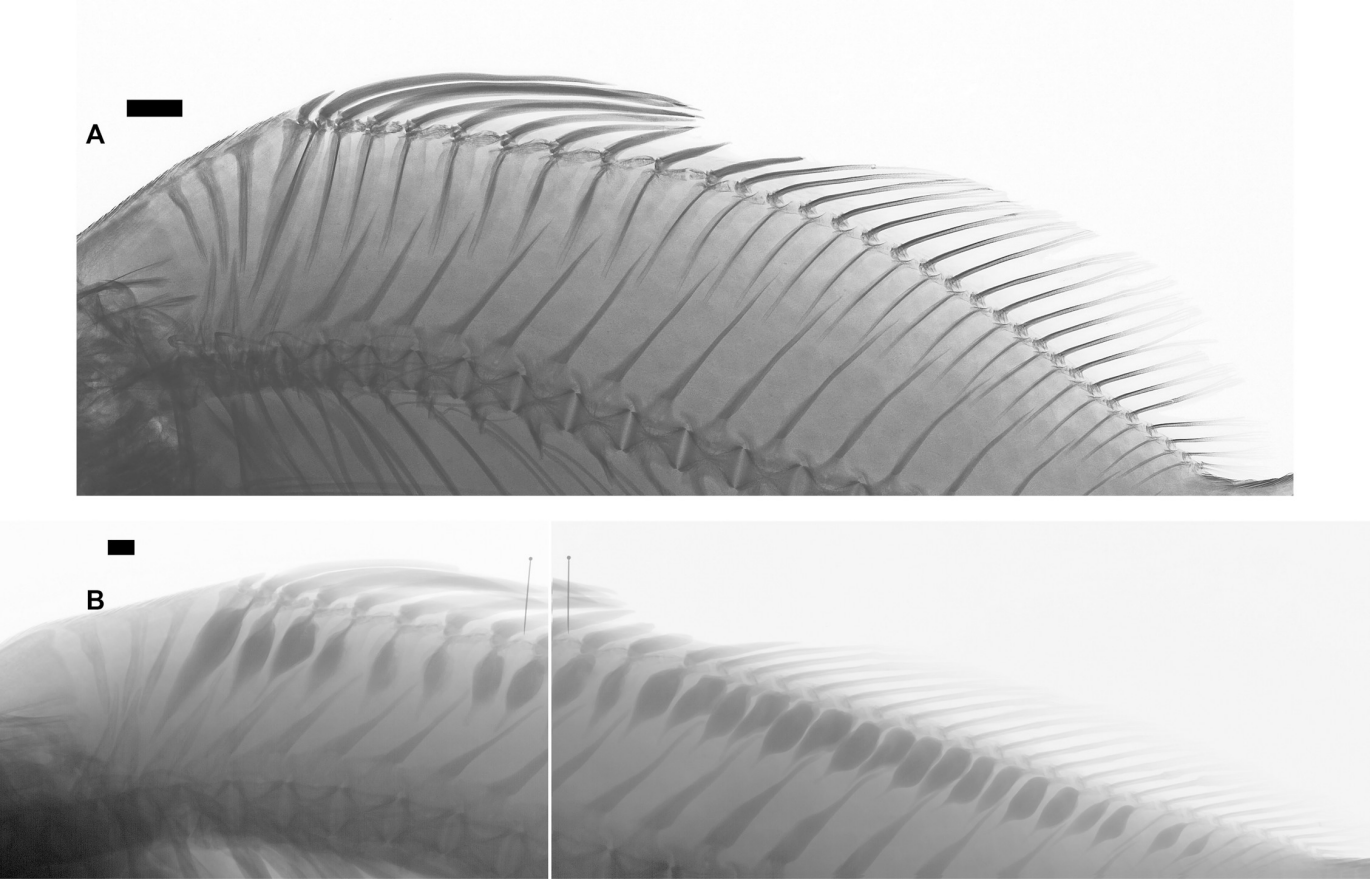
Pogonias cromis*. (A). USNM 142760, radiograph of an adult, about 300 mm SL. Note the absence of ray enlargements. (B). ANSP 162287, Radiograph of an adult, 720 mm SL. Note modifications in the size of dorsal-fin pterygiophores. Scale bars = 1 cm.
